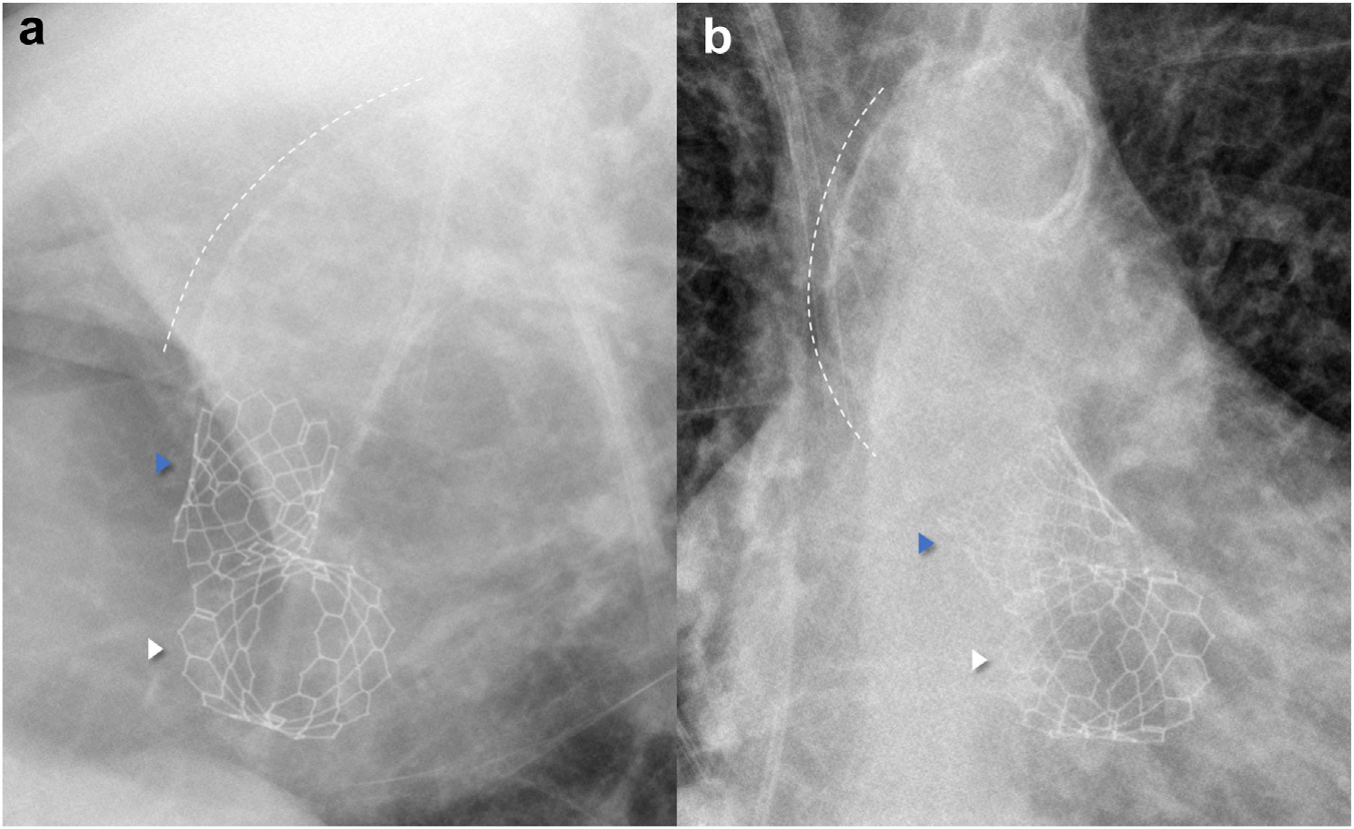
Porcelain aorta, transcatheter aortic and mitral heart valves. Lateral (a) and Anteroposterior (b) chest x-ray showing both SAPIEN S3 Ultra valves (Edwards Lifesciences, California, USA) in the aortic (blue arrowhead) and mitral (white arrowhead) positions, as well as the porcelain aorta (white dashed lines).
